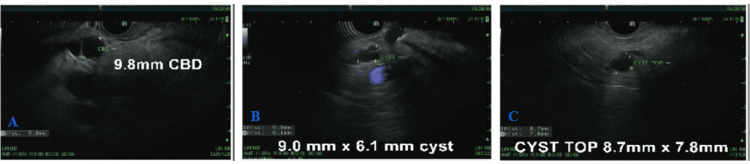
EUS showing CBD dilation to 9.8 mm (A). No stones, sludge, strictures, masses, or abrupt cutoffs were noted in the CBD; therefore, ERCP was not performed. Additionally, two anechoic cysts were found: one in the pancreatic head and the other in the pancreatic body, measuring 9.0 x 6.1 mm and 8.7 x 7.8 mm, respectively (B and C). EUS, endoscopic ultrasound; CBD, common bile duct; ERCP, endoscopic retrograde cholangiopancreatography
